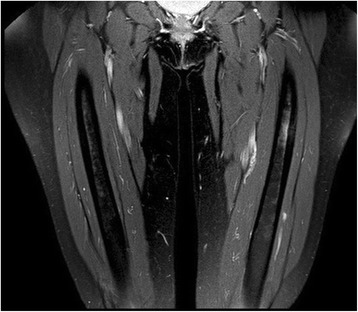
STIR-weighted MRI of the same case shown in Fig. 4. Note the increased fluid signal
